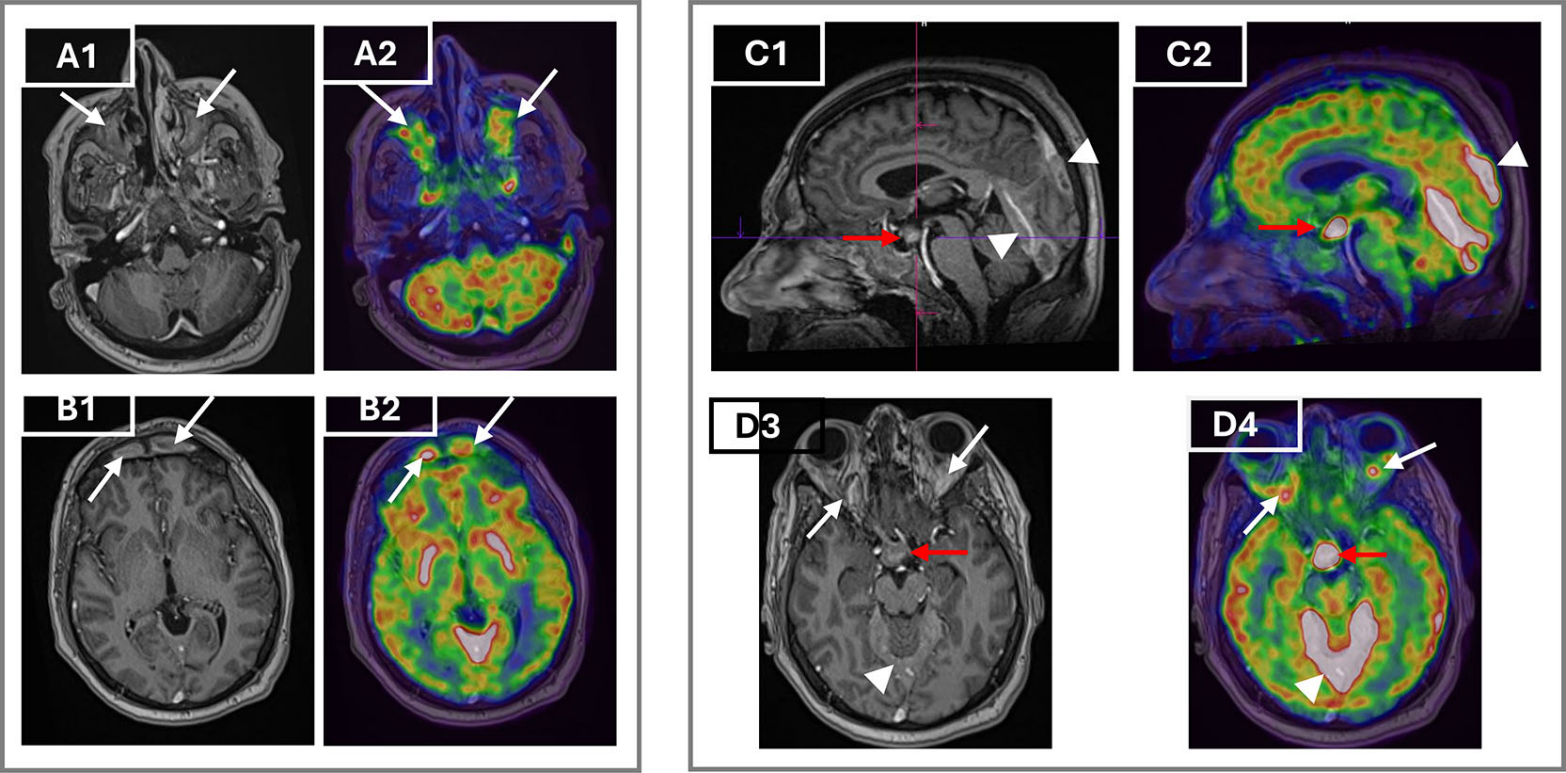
MRI and FDG PET/CT demonstrating orbital, craniofacial and central nervous system involvement in Erdheim–Chester disease before treatment. Axial post-contrast T1-weighted MRI (A1) and corresponding axial FDG PET/CT fusion image (A2) demonstrate intense enhancement and hypermetabolism of the maxillary sinuses (arrows). Axial post-contrast T1-weighted MRI (B1) and PET/CT (B2) show bilateral hypermetabolic frontal sinus involvement (arrows). Sagittal T1-weighted MRI (C1) and PET/CT (C2) reveal a suprasellar mass thickening the infundibulum (red arrows) and pachymeningeal enhancement of the tentorium cerebelli and adjacent meninges (arrowheads). Axial T1-weighted MRI (D3) and PET/CT (D4) show bilateral retroorbital masses (white arrows) encasing the optic nerves, along with hypermetabolism in the suprasellar mass (red arrows) and tentorium cerebelli (arrowheads). Abbreviations: MRI = magnetic resonance imaging;
